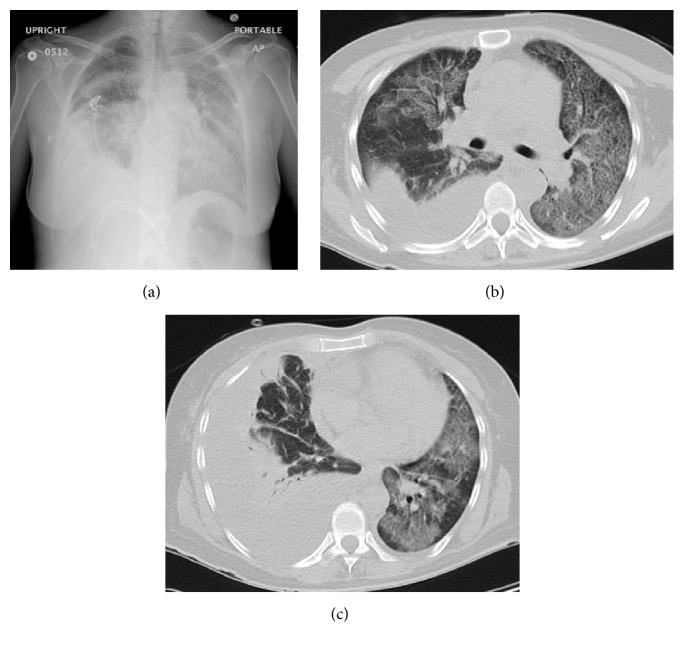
Chest X-ray (a) shows bilateral multilobar lung infiltrate with the appearance of loculated right pleural effusion. CT scan of the chest shows patchy airway disease throughout the upper lung lobes (b) as well as lower lung lobes (c).
